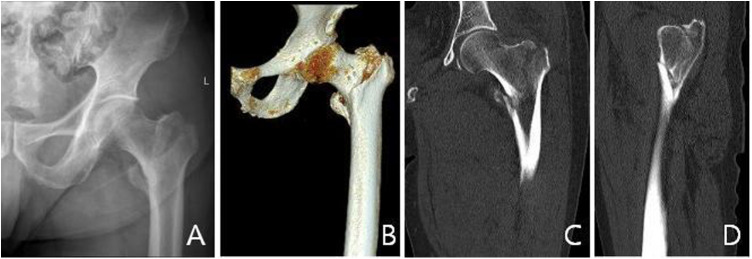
Preoperative radiographs and CT. (A) preoperative anteroposterior X-ray images of the left hip. (B) Three-dimensional CT reconstruction. (C) Coronal CT image. (D) Sagittal CT image.
